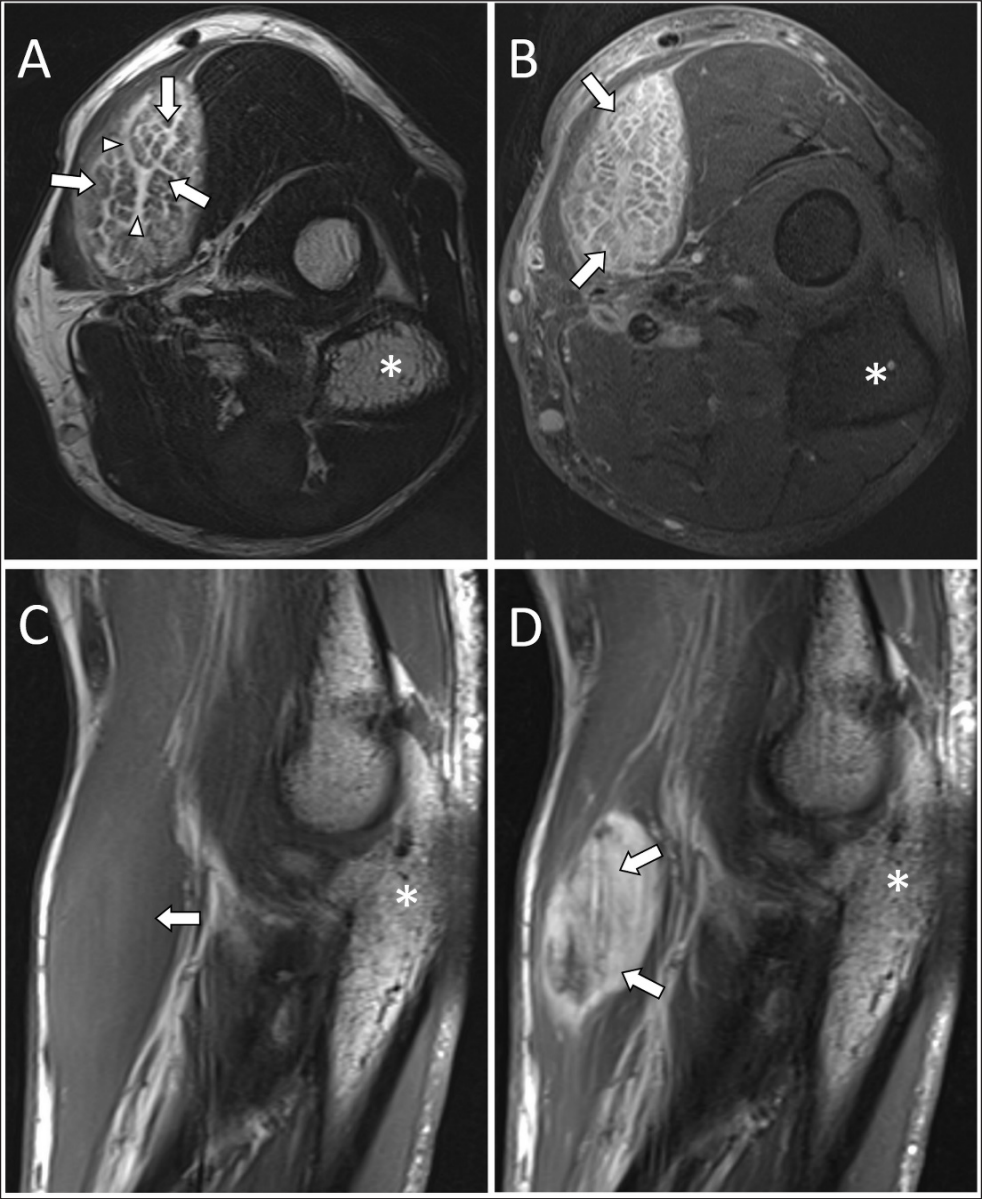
MRI images of PM in the left brachioradialis muscle. Axial T2WI (A), axial post-contrast T1WI fat-saturated (B), sagittal T1WI (C), and sagittal post-contrast T1WI (D) images demonstrate a T2 hyperintense lesion with a marked enhancement. The T2WI (A) shows hyperintense bands (white arrowheads) interspersed with intact muscle fibers (white arrows). Olecranon (*).
